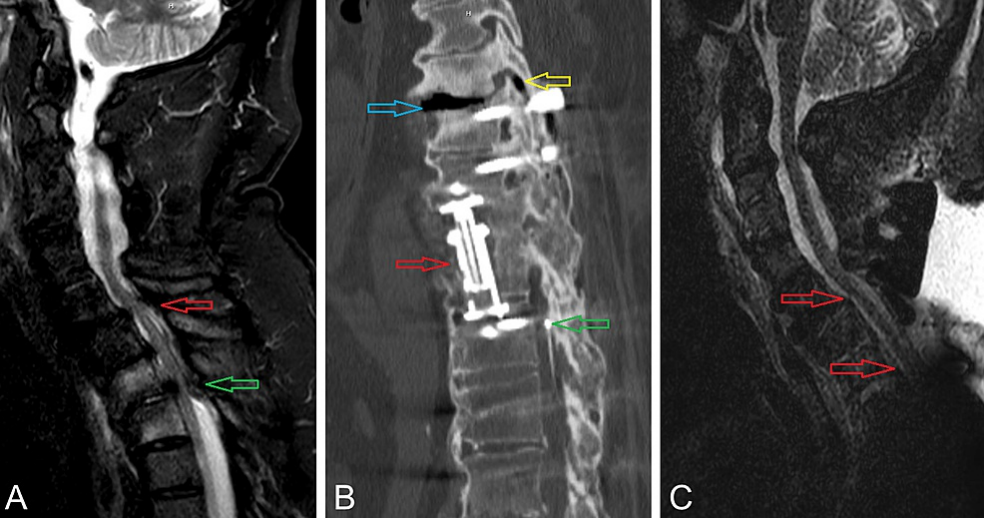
(A) Preoperative MRI T2 sagittal view demonstrates C7-T1 severe stenosis (red arrow) related to adjacent segment disease given the history of prior C3-C7 fusion. Severe central stenosis is also demonstrated at the T2-T3 level (green arrow). (B) Sagittal view of a CT study demonstrating prior T9-T10 corpectomy cage (red arrow) with T8-L2 fusion. The intrathecal catheter tip is visible, terminating within the spinal canal at the level of the T11 pedicle (green arrow). Air is also visible within the T7-T8 disc space (blue arrow) and the T7-T8 facet joint (yellow arrow), indicating the presence of significant mobility at that level. (C) Postoperative MRI T2 sagittal view demonstrating decompression of the C7-T1 and T2-T3 segments with the restoration of cerebrospinal fluid signal ventral to the spinal cord (red arrows).
